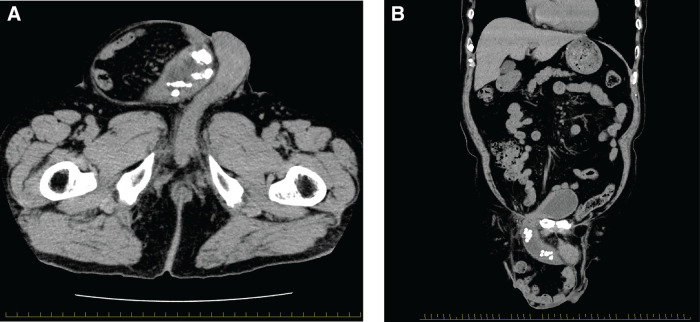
(A) CT image of a bladder stone in the herniated bladder, coronal section. (B) CT image of a bladder stone in herniated bladder, axial section.
